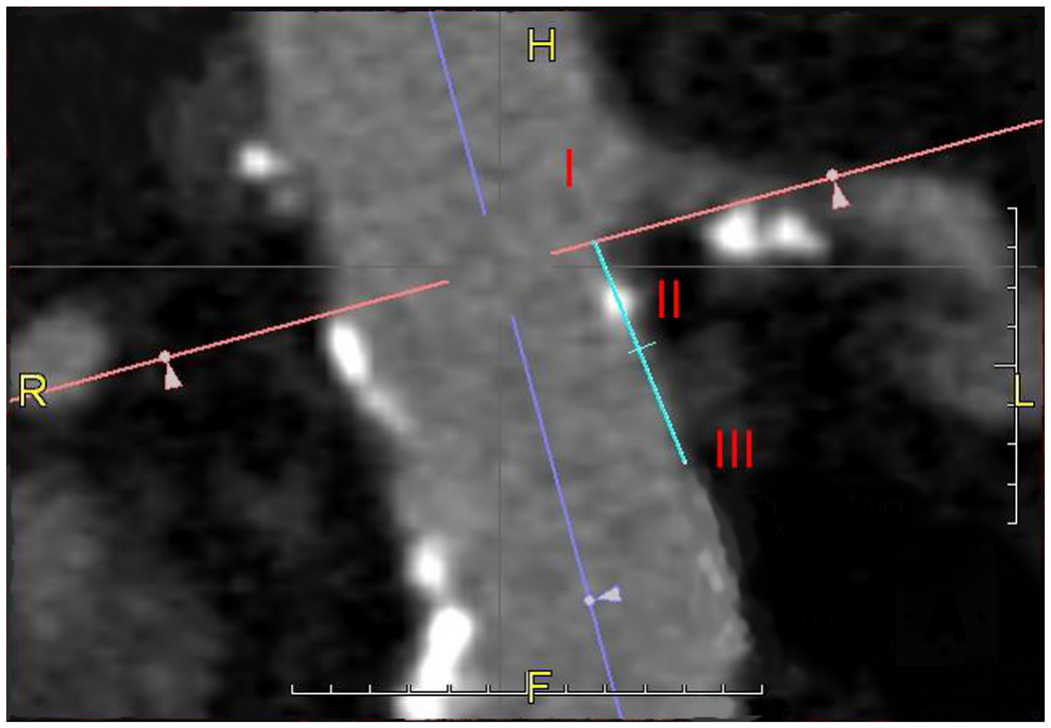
Computed tomography image of the abdominal aorta. In Roman numerals the infra renal distances are portrayed. I: 0 mm, II: 7.5 mm, and III: 15 mm. To provide a standardized method for measuring the distances as mentioned above, first the midline of the aortic neck is chosen. The perpendicular line is then placed at the point where the lowest renal artery branches from the aorta. In the caudal direction from this point (I) and parallel to the midline, a distance of 7.5 and of 15 mm is measured to identify point II and III, respectively.
